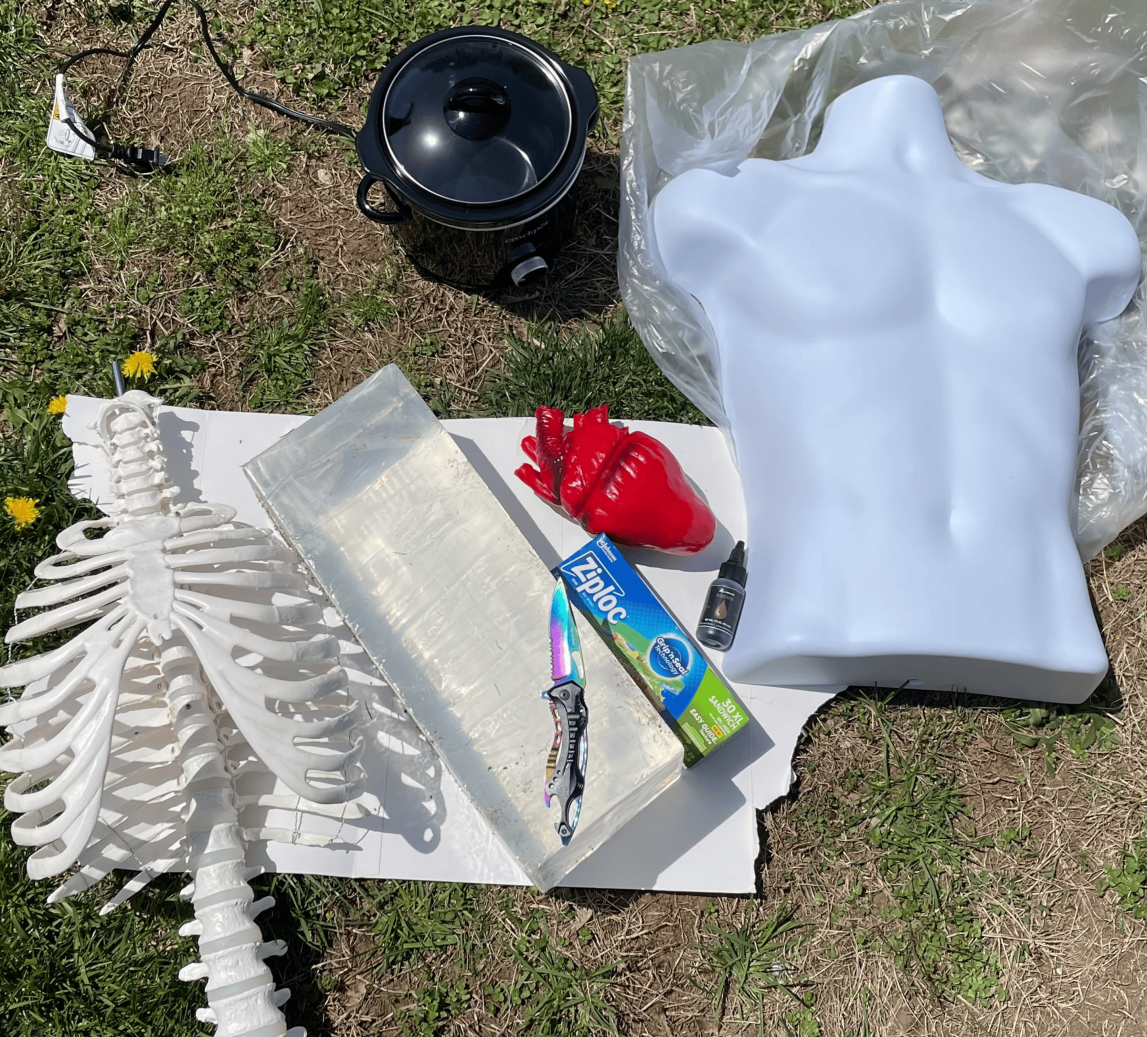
Component Materials For Model Making Please note that, due to poor echogenicity, the foam heart shown in this photo was not used in the final model. The heart was instead replaced by ping pong balls and a plastic ultrasound probe cover not shown here.
